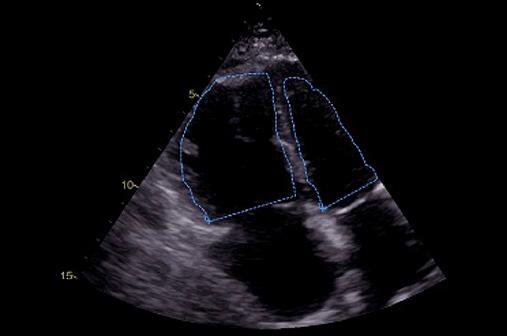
Função sistólica do VD	Hipocinesia ou acinesia de paredes. FAC < 35%. TAPSE < 17 mm. *Doppler* tecidual: onda S’ < 9,5 cm/s. “ *Strain* ” longitudinal de parede livre acima de -20% (< 20% em valor absoluto). Eco 3D: fração de ejeção < 45%.
